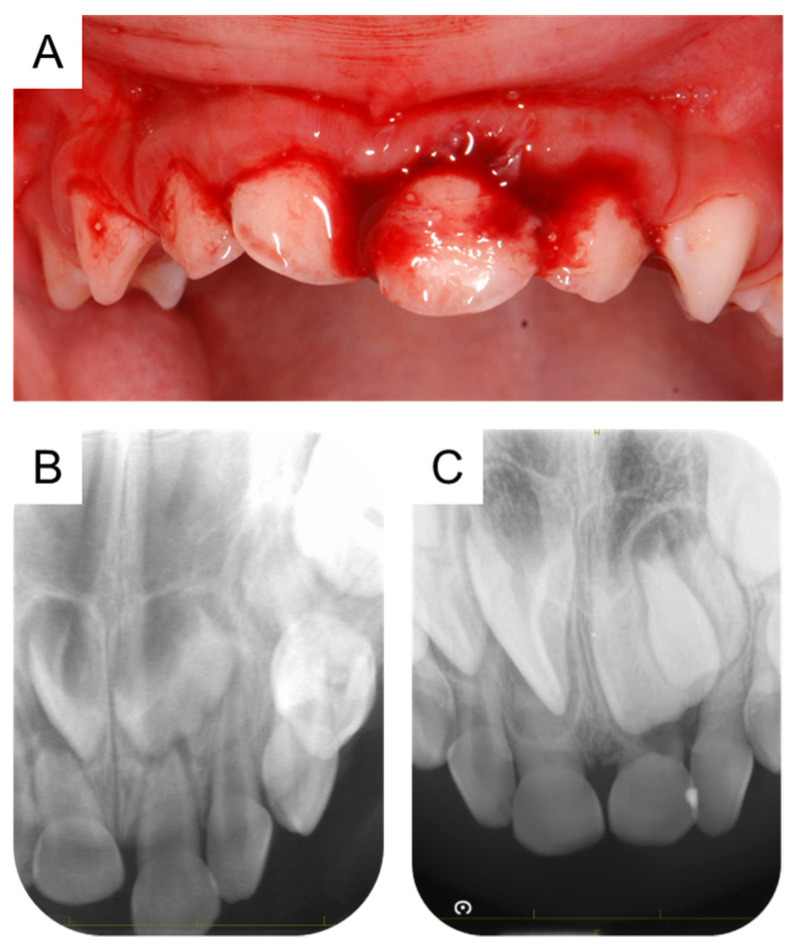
Intraoral photograph and periapical radiographs before the eruption of the maxillary permanent incisors. (A,B) Initial examination at the age of 2 years and 1 month. (C) Periapical radiograph at the age of 6 years and 5 months.
